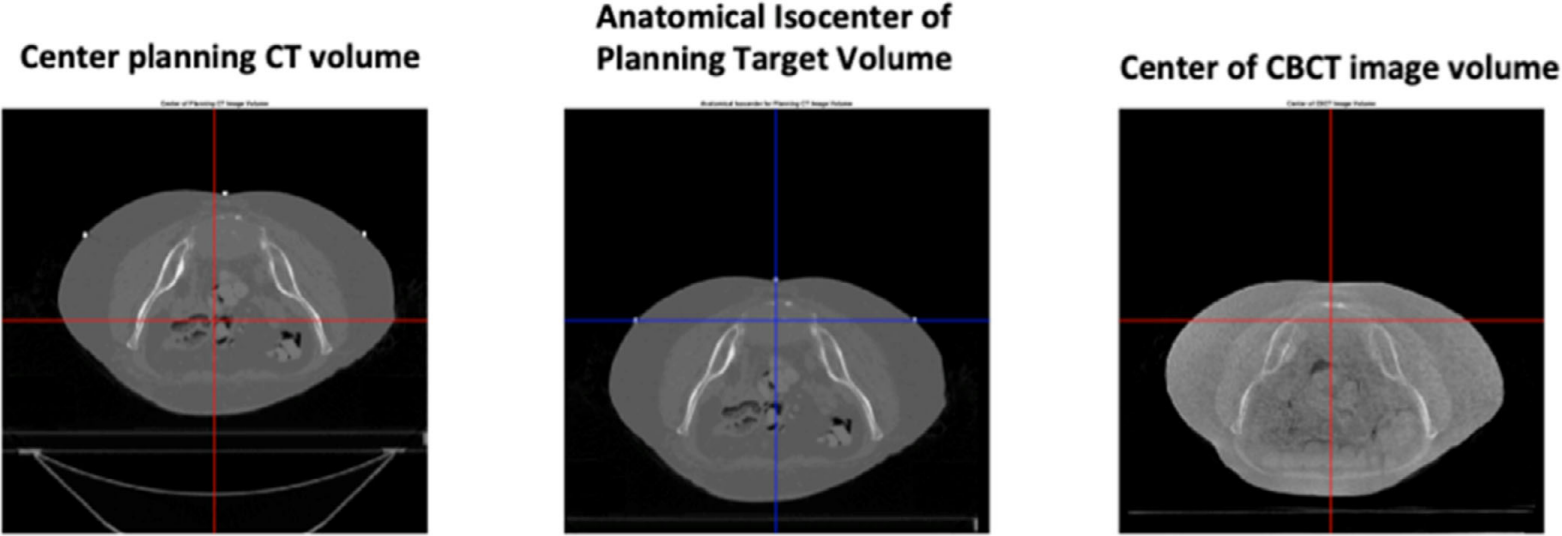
Planning computed tomography (CT) and cone beam CT images.
